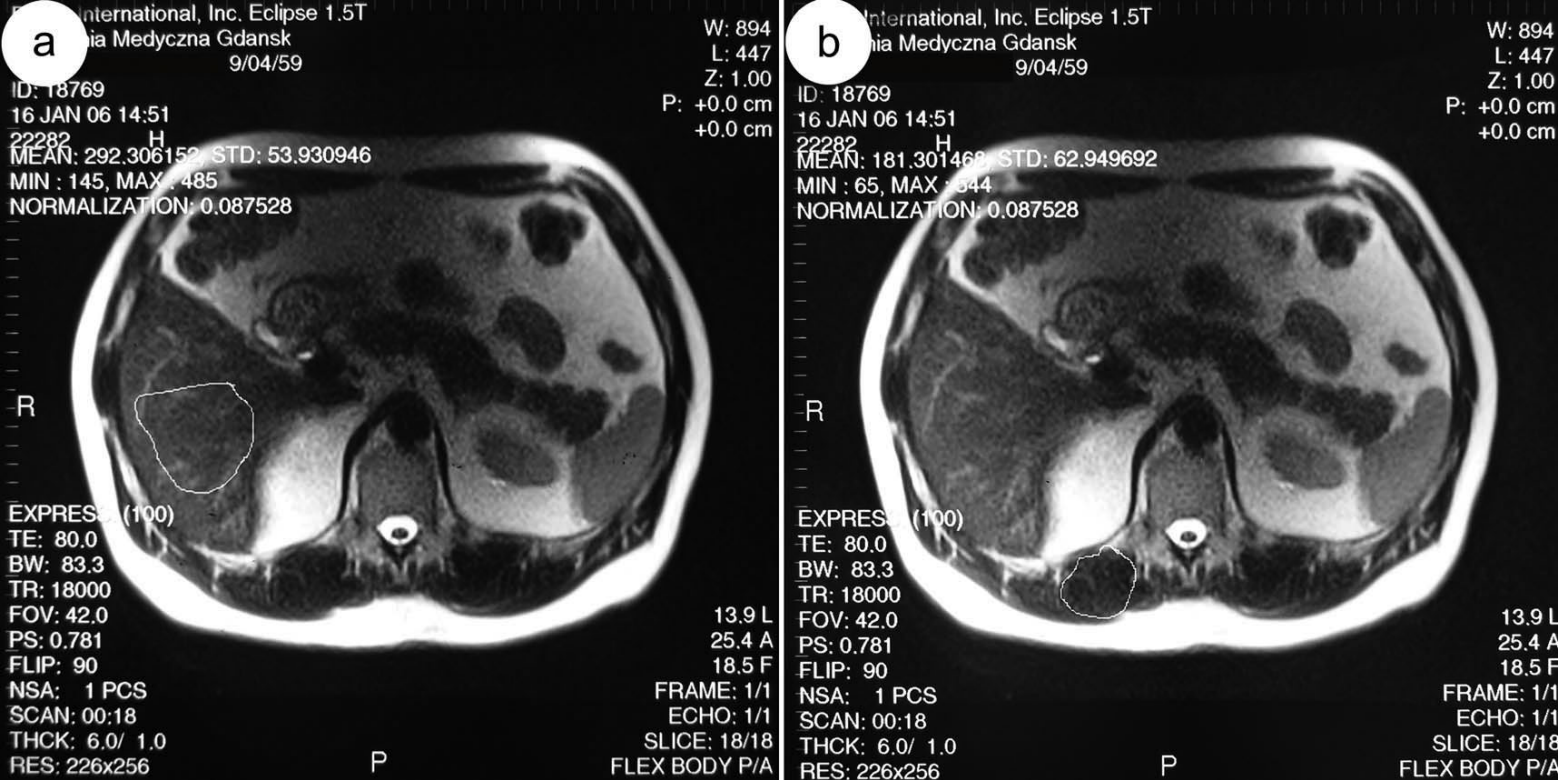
47-year-old man with normal hepatic iron level confirmed by the biopsy. Axial MR T2-weighted images performed on gradient echo sequence show normal liver. The signal intensity of liver (fig.1a - the signal intensity of liver is 292) is higher than that of paraspinal muscles (fig.1b - the signal intensity of paraspinal muscles is 181).
